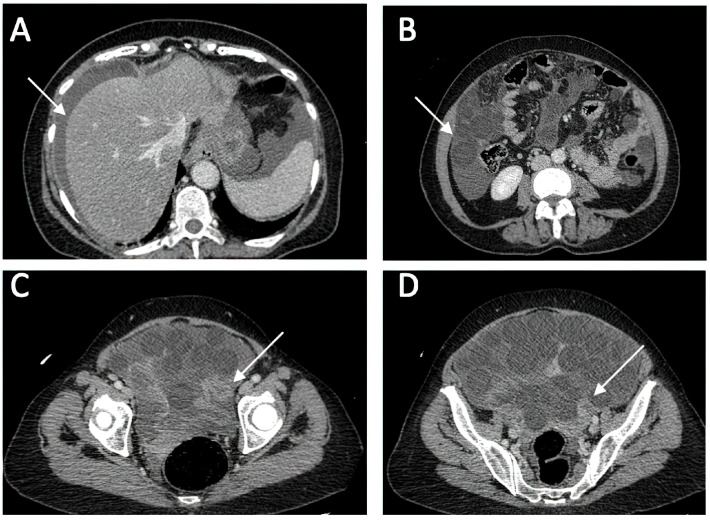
Diagnostic CT Imaging of Abdominal and Pelvic Regions. (A) ascites (B) multiple round intra-peritoneal fatty density lesions without contrast uptake; (C,D) serpiginous structure with intense contrast uptake at the level of the left ovary.
